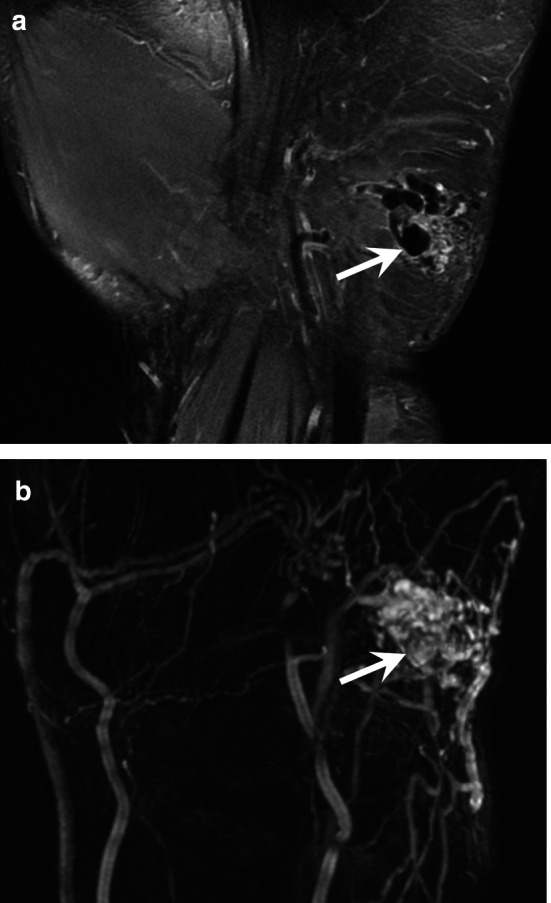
AVM of the hypothenar eminence in a 37 year-old-woman. a Coronal T2-weighted MR image showing the nidus with multiple enlarged vessels with flow void (arrow) indicating a high-flow malformation. b MRA demonstrating the high flow of the malformation with a rapid vascular enhancement of the nidus (arrow), multiple feeding arteries, and early venous drainage through dilated veins
